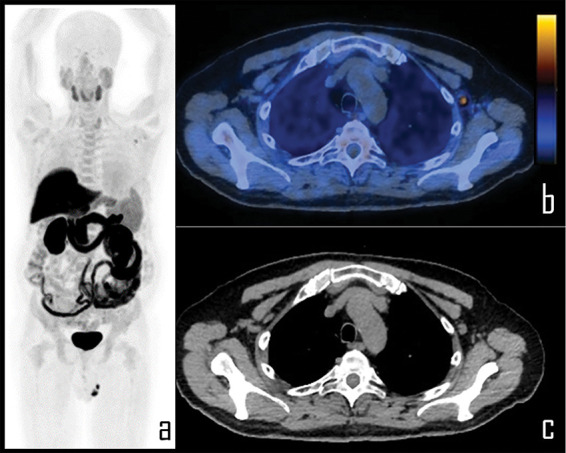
A 65-year-old prostate cancer patient underwent [^18^F]Choline PET/CT due to biochemical relapse of disease, two years after radical prostatectomy. PSA at the time of the scan was 0.4 ng/mL. Patient also underwent COVID-19 vaccine injection in the left arm 6 days before the exam. Note: PET imaging (a) was negative for disease relapse but showed a single area of focal [^18^F]Choline uptake in the left axilla, corresponding to a 1 cm wide, ovoid lymph node, displayed in axial PET/CT view (b), with fatty hilum detectable in the axial, co-registered *low dose* CT (c). This lymph node was considered as reactive, following vaccine administration; SUVmax was 2.3.
